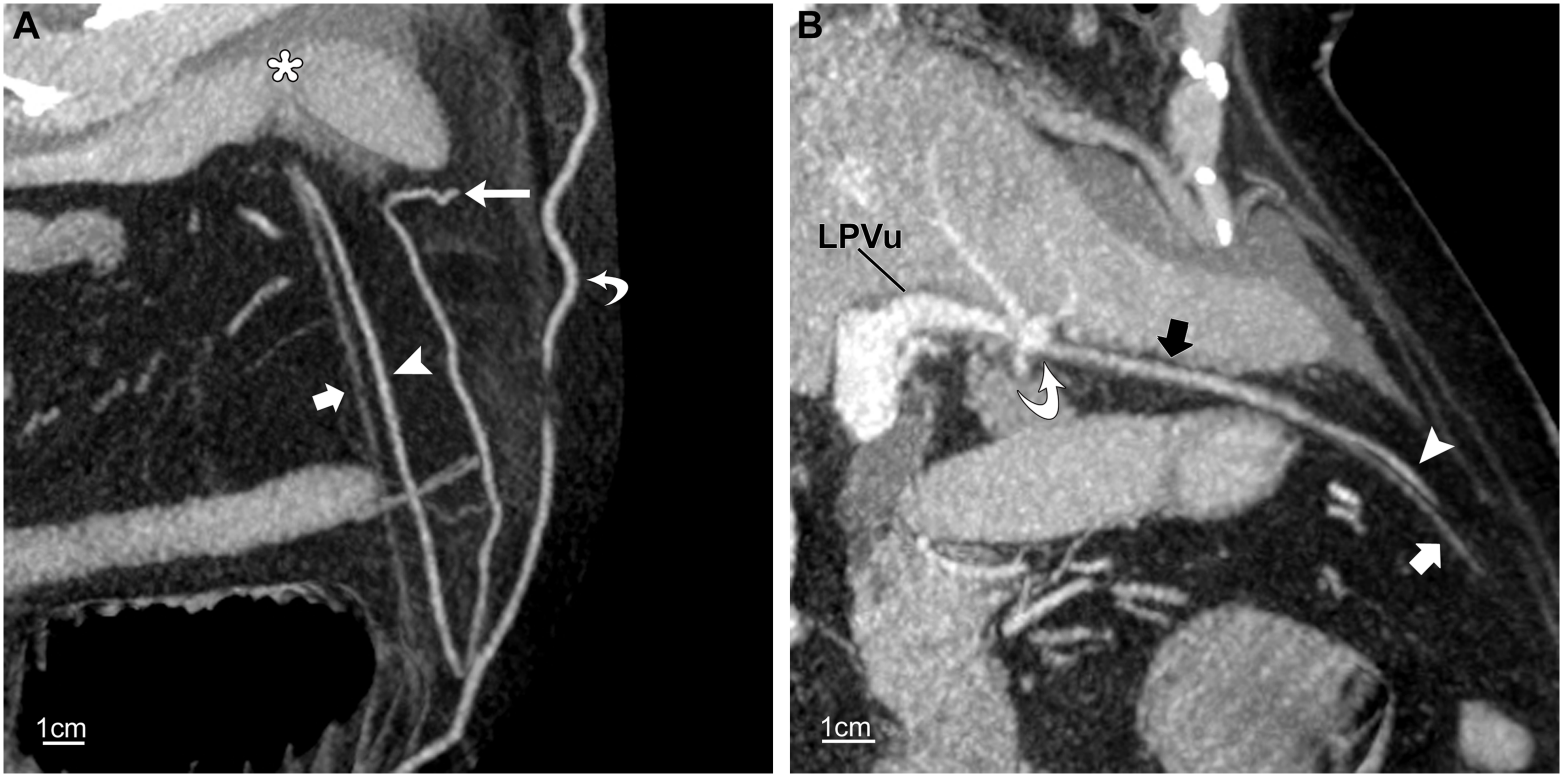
Inferior paraumbilical vein. Routine contrast-enhanced CT of abdomen in a 69-year-old woman with SVC obstruction. (A, B) Oblique sagittal 7-mm maximum-intensity-projections in different planes show an inferior paraumbilical vein (arrowheads), fed by an ensiform vessel (long arrow, A), draining into the umbilical vein (short arrows) just before the liver (asterisk, A). The opacified umbilical vein (black arrow, B) enters the recessus umbilicalis (curved arrow, B) of the left portal vein (LPVu). The umbilical vein below the union (short white arrows) is smaller and not opacified. Also shown is a collateral subcutaneous vein (curved arrow, A).
